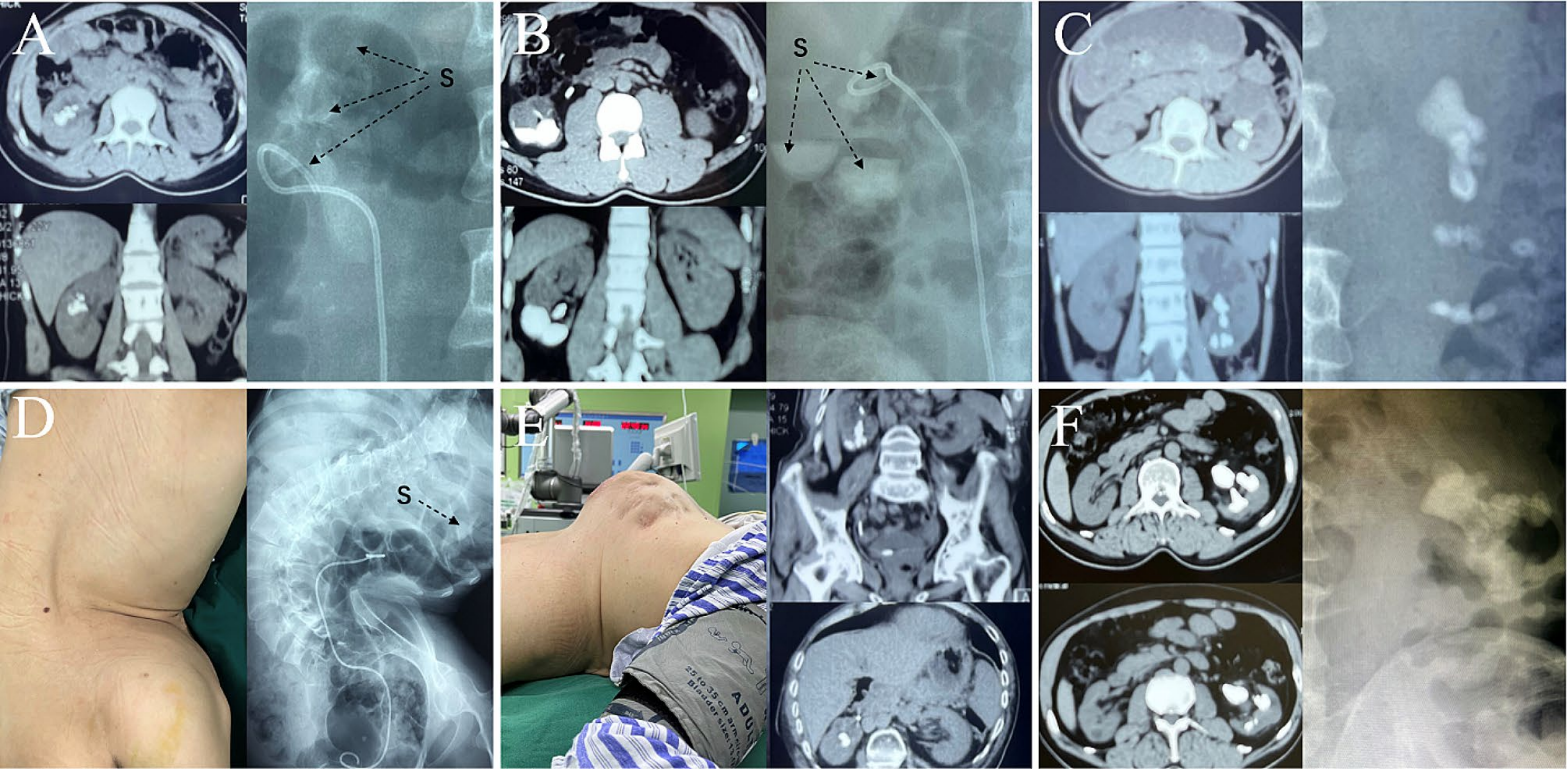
The preoperative abdominal non-contrasted computed tomographic (NCCT) and plain kidney-ureter-bladder (KUB) films of six enrolled cases with complex kidney stones (CKS). (A) Case 3, a partial staghorn stone with multiple stones in medium calyceal diverticulum, was assessed as Guy’s Stone Scores (GSS) grade III; (B) Case 11, multiple stones in the renal pelvis and lower calyceal diverticulum, was assessed as GSS grade III; (C) Case 8, a partial staghorn stone with multiple stones in lower calyceal diverticulum, was assessed as GSS grade III; (D) Cases 6, a stone in the pelvis of a solitary ectopic thoracic kidney (ETK) with a severe congenital scoliosis deformity, was assessed as GSS grade IV; (E) Case 9, multiple stones in the pelvis and lower calyceal diverticulum of an ETK with a severe rachiterata caused by spinal tuberculosis, was assessed as GSS grade IV; (F) Case 4, a complete staghorn stone, was assessed as GSS grade IV. Unclear high-density shadows of stones are denoted by black arrows in plain KUB films. S = stone
